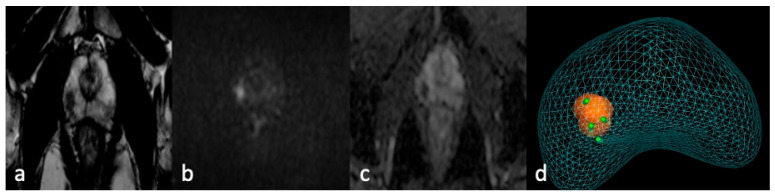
MRI-TBx procedure. Apex right PZpl prostate lesion at mpMRI (PI-RADS 4): (a) MRI-T2 image; (b) MRI-DWI image; (c) MRI-ADC image; (d) MRI-targeted biopsy report.
